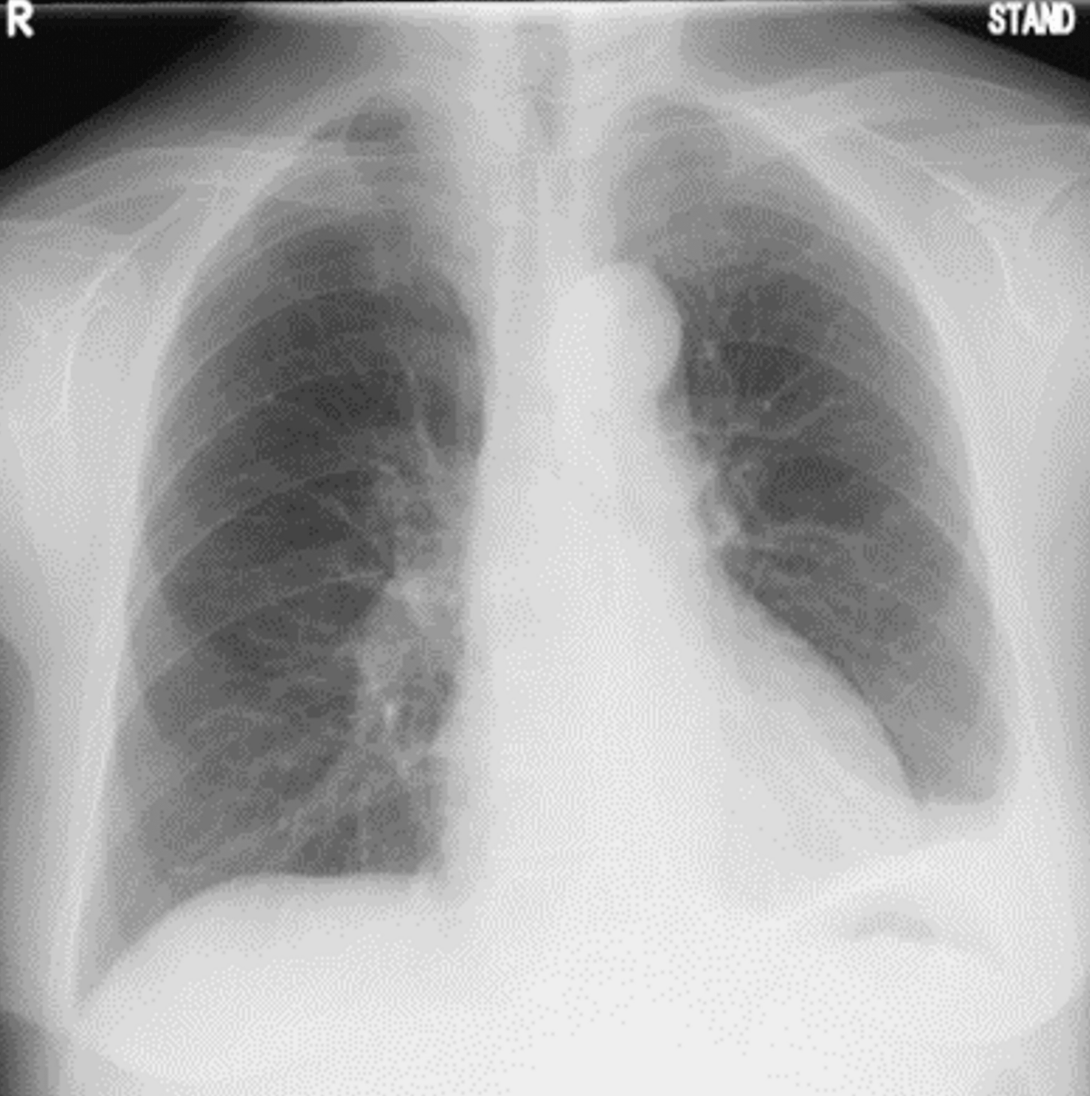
Radiography on the 13th hospital day The pleural effusion in the right lung has disappeared.
